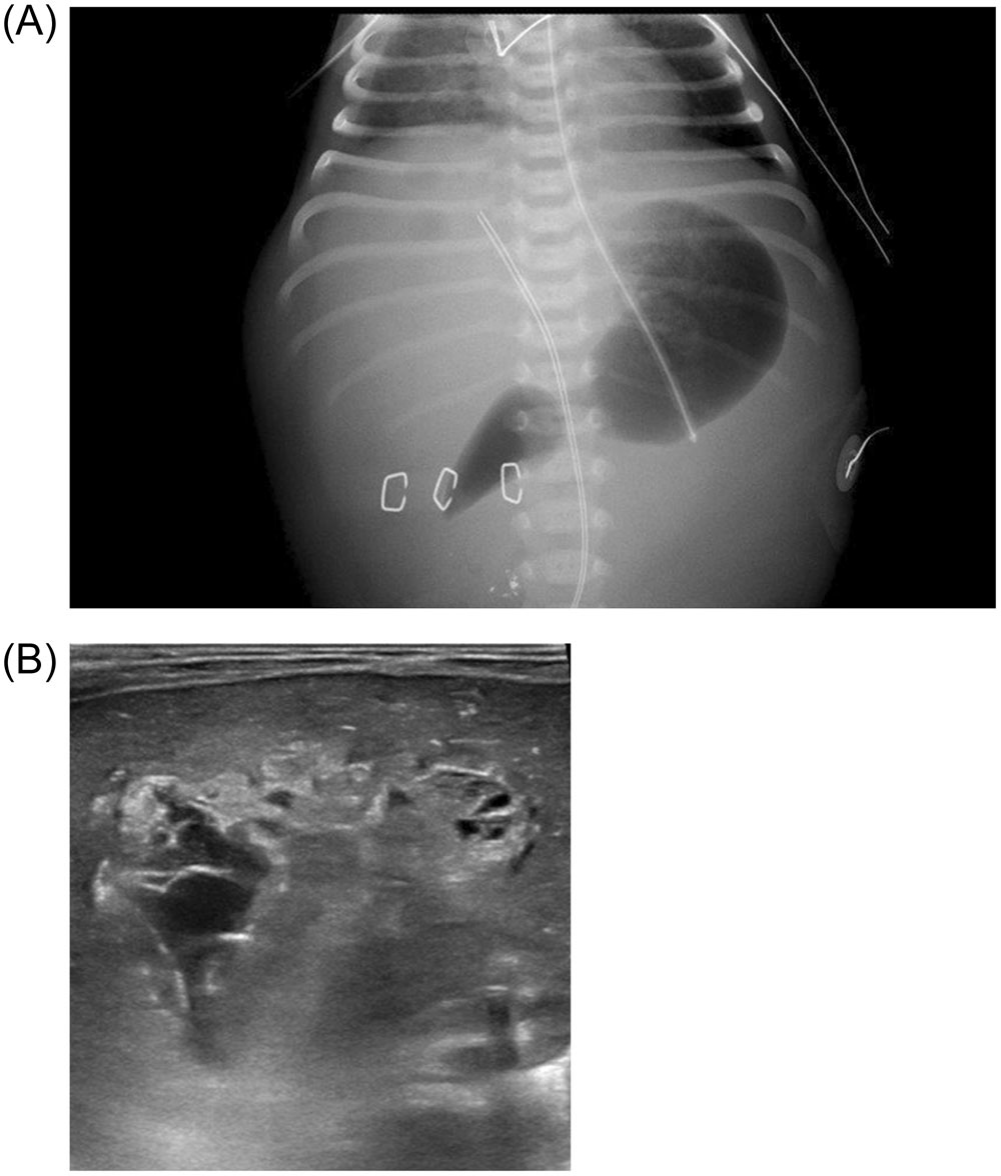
Hepatic collection findings on X‐ray (A) and sonography (B).
